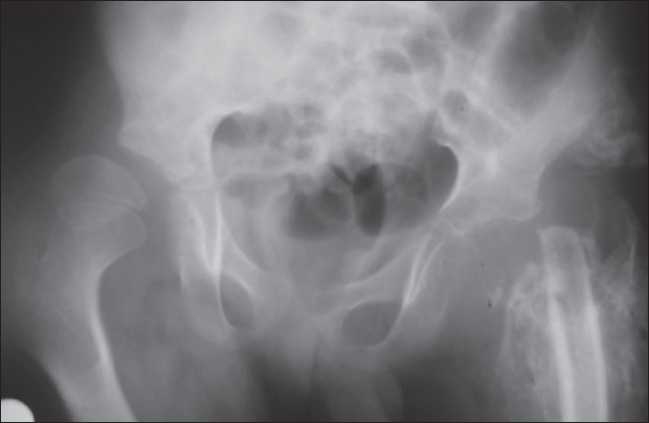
X-ray examination shows necrosis, osteomyelitis, and abscess formation in the left caput femoris
